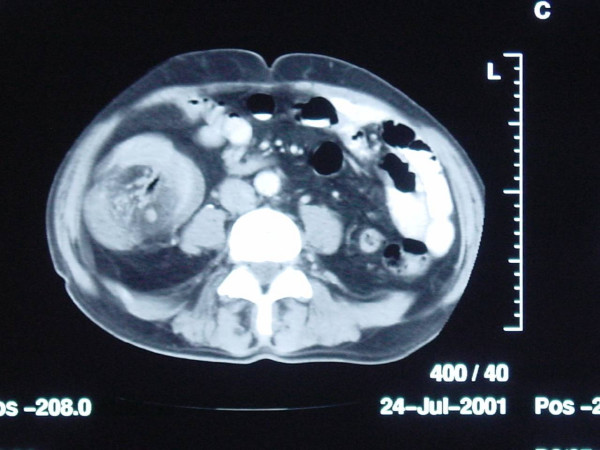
CT scan of abdomen. Red arrow demonstrates a "target lesion" diagnostic of intussusception.
